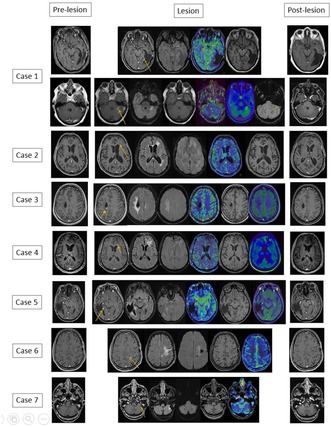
FIGURE 1 Representative MRI/PET images of the seven reported cases before, during, and after LPTE (Late transient post‐radic enhancements).
